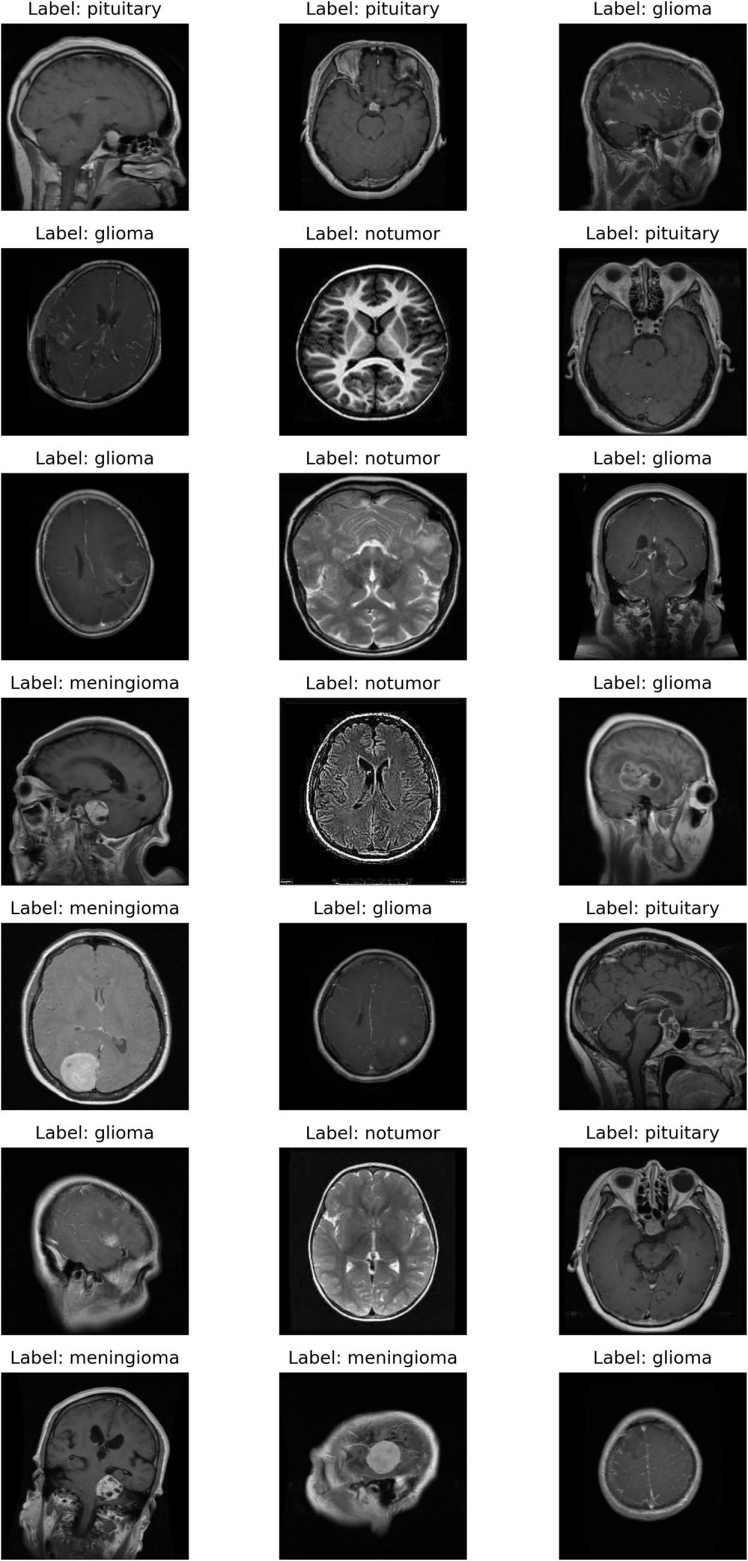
The labeling of the MRI images used in this study varied in both orientation (horizontal, sagittal) and type (T1, T2)
